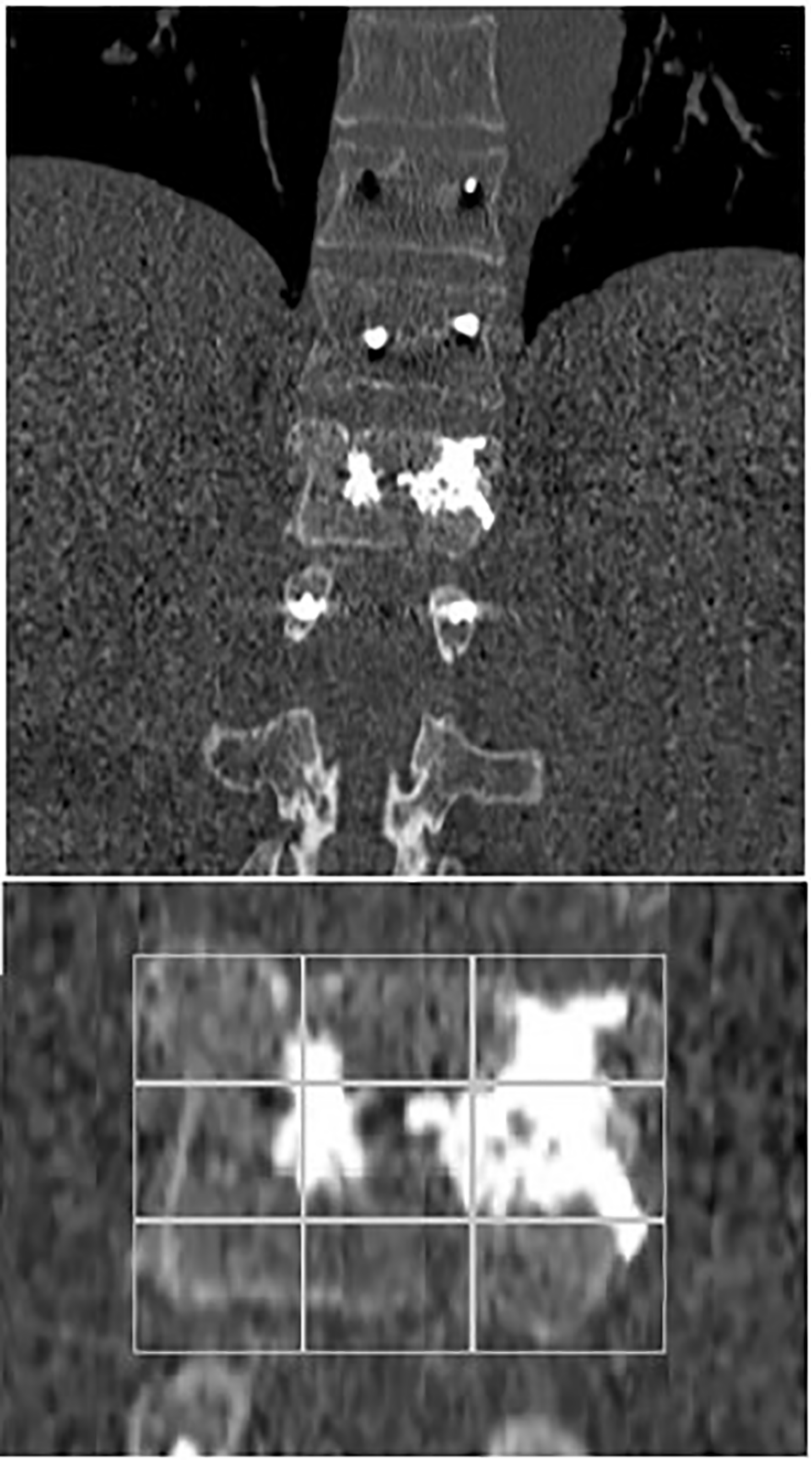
Coronal early post-operative CT scan showing an L1 average cement filling based on Saliou filling score 11/18 (6 + 5) and a T11-T12-L2 vertebral fixation.
